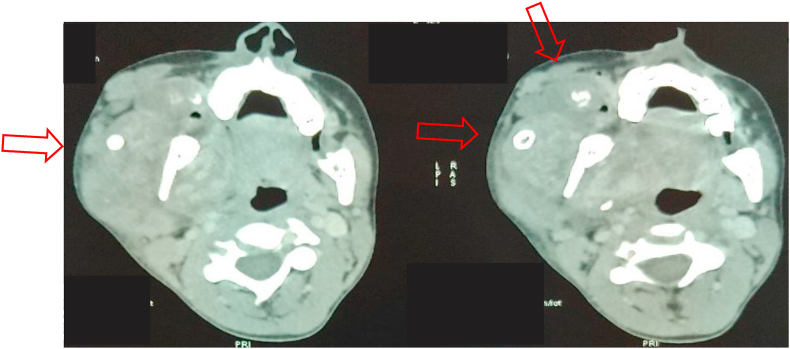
CT scan showing vascular malformation with macro calcifications.
